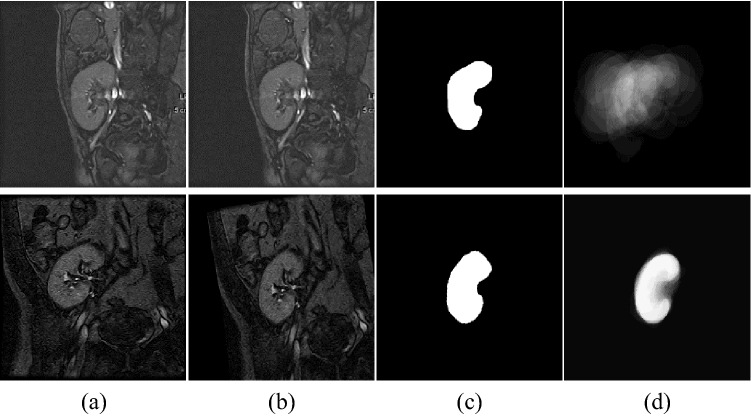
Kidney shape model construction from DCE-MRI images: (a) Sample images, (b) Images after affine transformation, (c) Segmented kidneys after alignment, (d) The shape prior model constructed using the Bayesian parameter estimation method before (top) and after (bottom) the affine registration.
